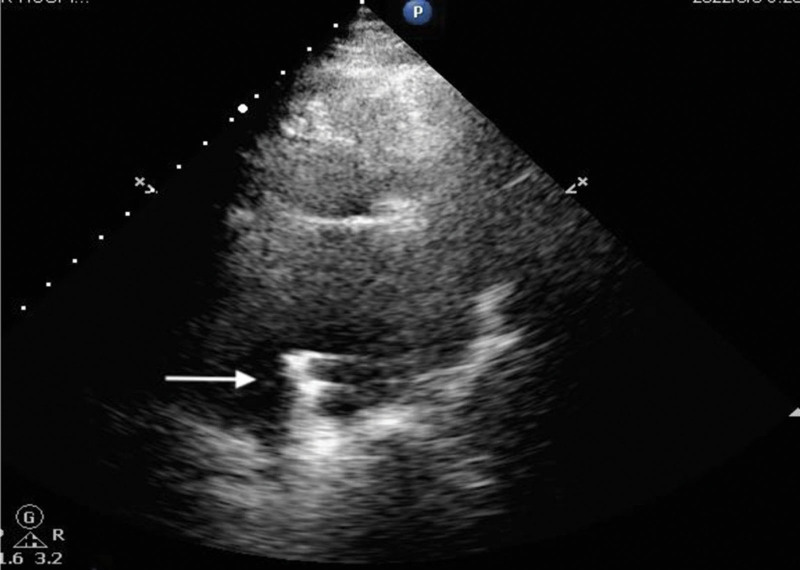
TTE displayed strong echogenic oscillations in the left atrium. TTE = transthoracic echocardiography.
